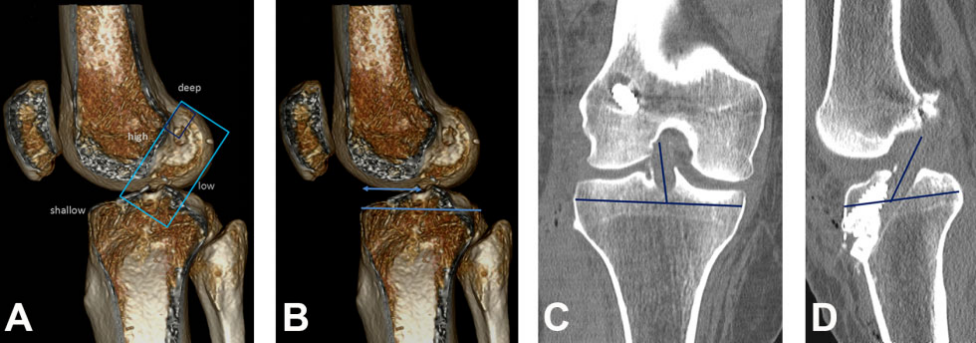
(A) Femoral tunnel measurement according to Bernard and Hertel, as depicted on 3-dimensional computed tomography (CT) after reconstruction with a hamstring graft. The graft tunnel center is 27% in the femoral deep-shallow direction and 35% in the femoral high-low direction (anatomic placement). (B) Tibial tunnel measurement according to Stäubli and Rauschning.^42^ The graft tunnel center is 46% in the tibial anterior-posterior direction (anatomic placement). (C) Coronal angle measurement on CT (example of reconstruction with a bone–patellar tendon–bone graft), measured at 80° (nonanatomic placement). (D) Sagittal angle measurement, measured at 60° (nonanatomic placement).
